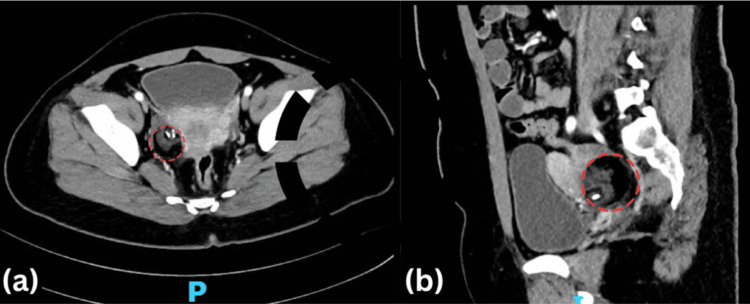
Lower abdominal CT scan (a) Axial view and (b) sagittal view showed a well-defined, fat-attenuating ovarian mass with intralesional calcifications and soft tissue densities measuring 2.6 x 3.2 x 3.9 cm.
